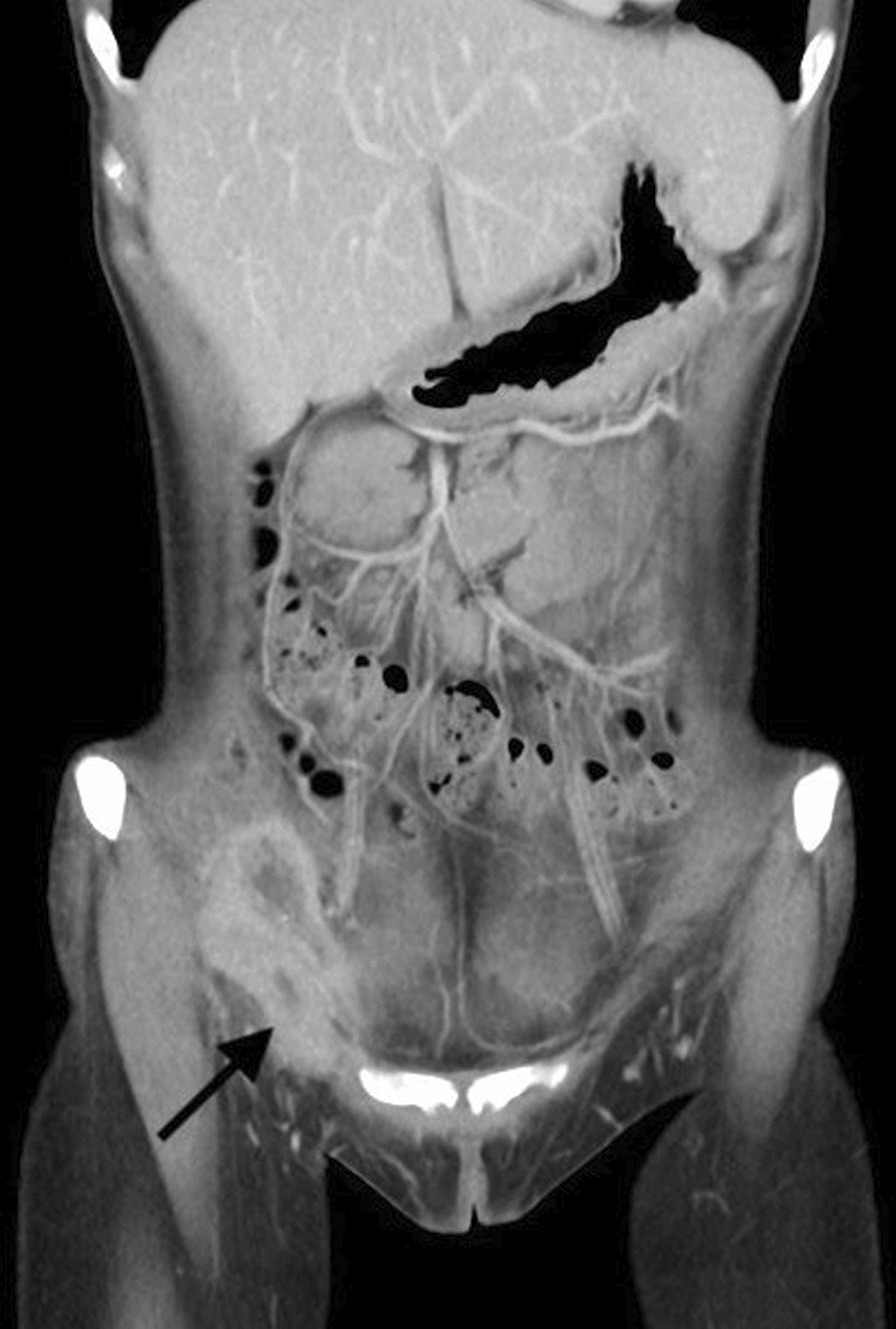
The follow-up contrast-enhanced abdominal CT revealed interval progression of the right lower abdominal abscess with transcompartment involvement, including the preperitoneal region and right inguinal canal, highly suspicious of incarcerated AH, secondary to ruptured appendicitis. (Arrow: Transcompartment inflammation into right inguinal canal)
